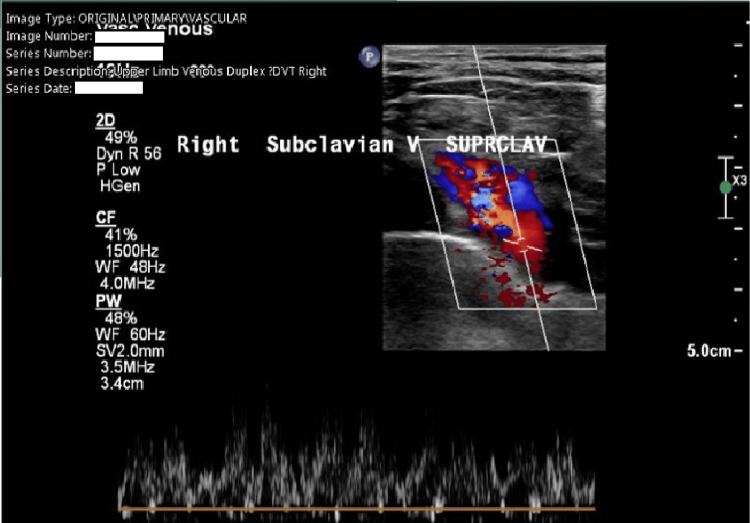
Ultrasound scan of the right upper limb showing the supraclavicular subclavian vein with an echogenic thrombus, as reported by the ultrasound technician.
